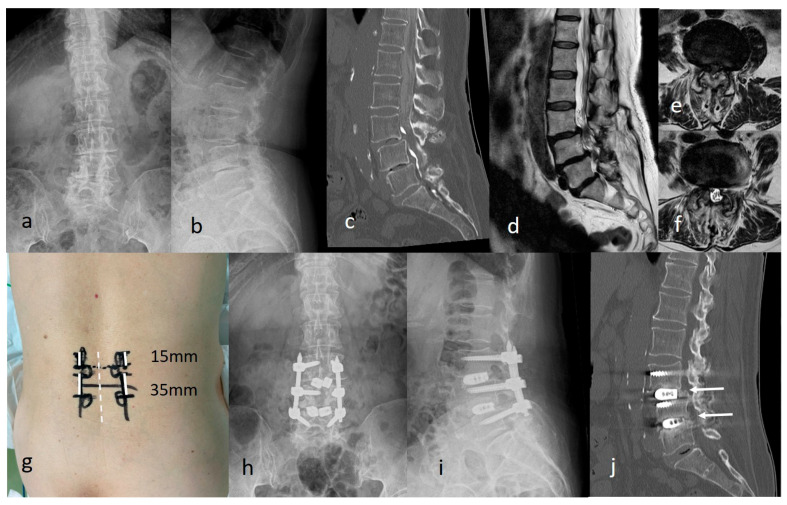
Revision case after lumbar decompression at L3/4 and L4/5. (a,b) Preoperative radiograph. (c–f) Preoperative computed tomography and magnetic resonance images showing spinal canal stenosis at L3/4 and L4/5. (g) The procedure is performed through four bilateral incisions (white solid line) without entering the spinal canal at the site of the previous surgery (white dotted line). (h,i) Postoperative radiograph. (j) Intervertebral bone fusion was achieved posterior to the cage at both levels (white arrows).
